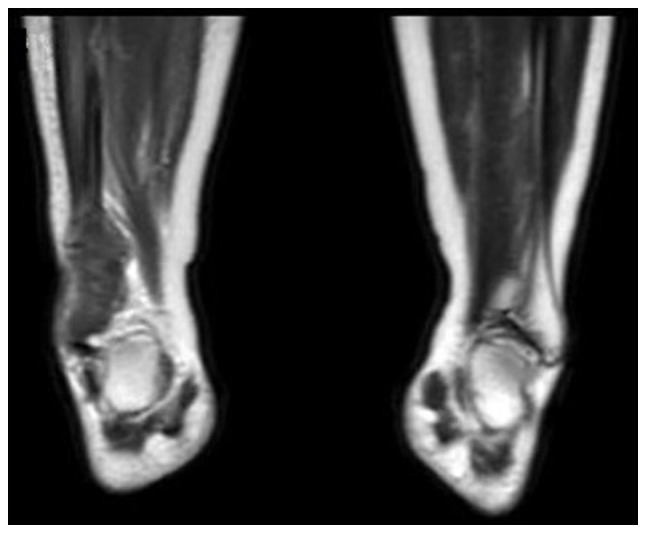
Magnetic resonance imaging of the ankle coronal section: Intense hypo tissue is observed in the distal portion of the right fibula, evidencing extension to soft tissues.
